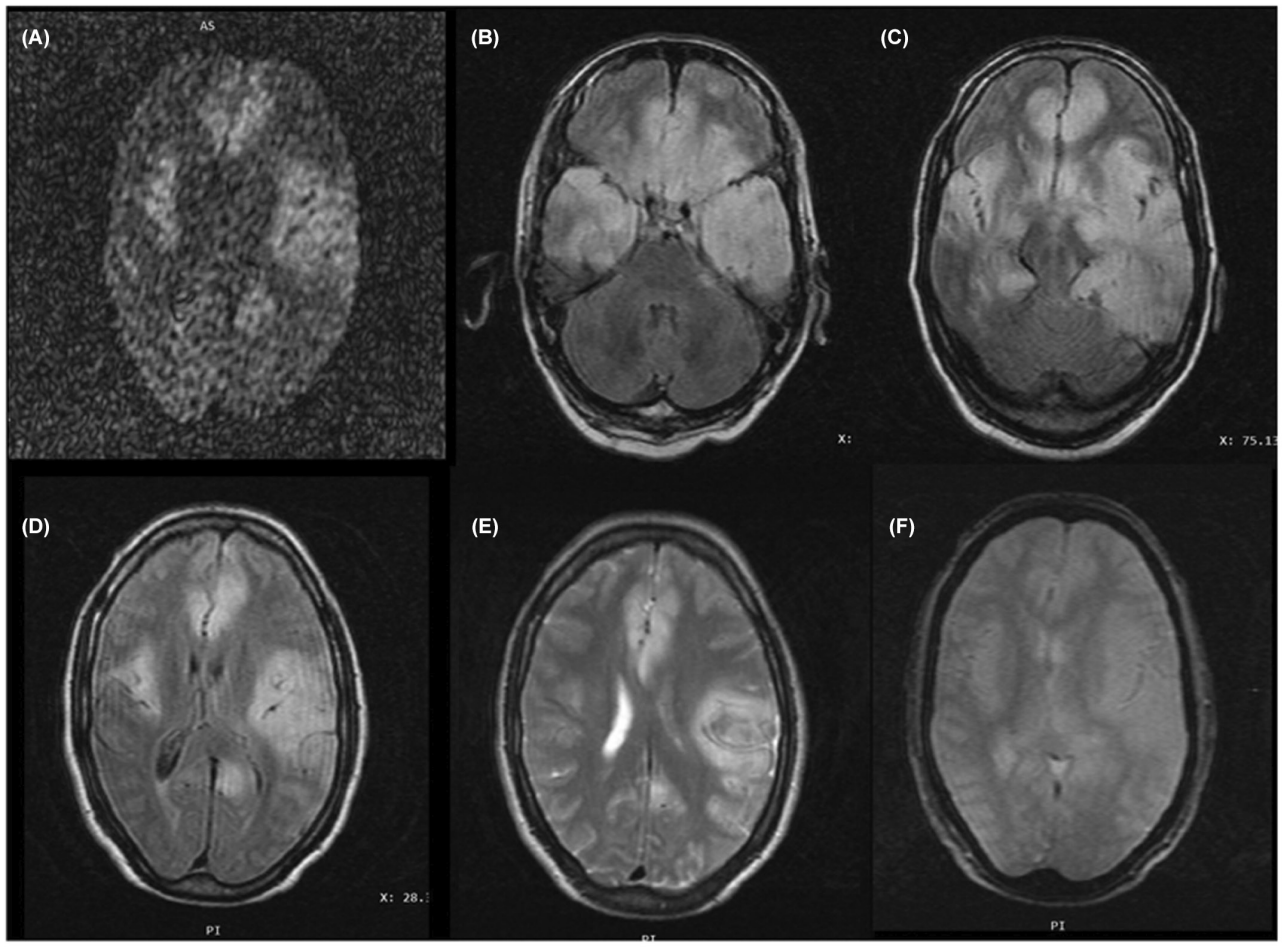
Brain MRI showed abnormal signal, T2/FLAIR (fluid attenuated inversion recovery) sequence showed hyperintensities, T1 sequence showed hypo‐intensities with symmetrical involvement of bilateral medial temporal lobes, bilateral external capsule, basal ganglia, subcortical part of frontal lobes and basifrontal lobes. GRE (Gradient echo) sequences did not show hemorrhages, hence acute hemorrhagic encephalomyelitis which is a form of ADEM was ruled out (A–F).
